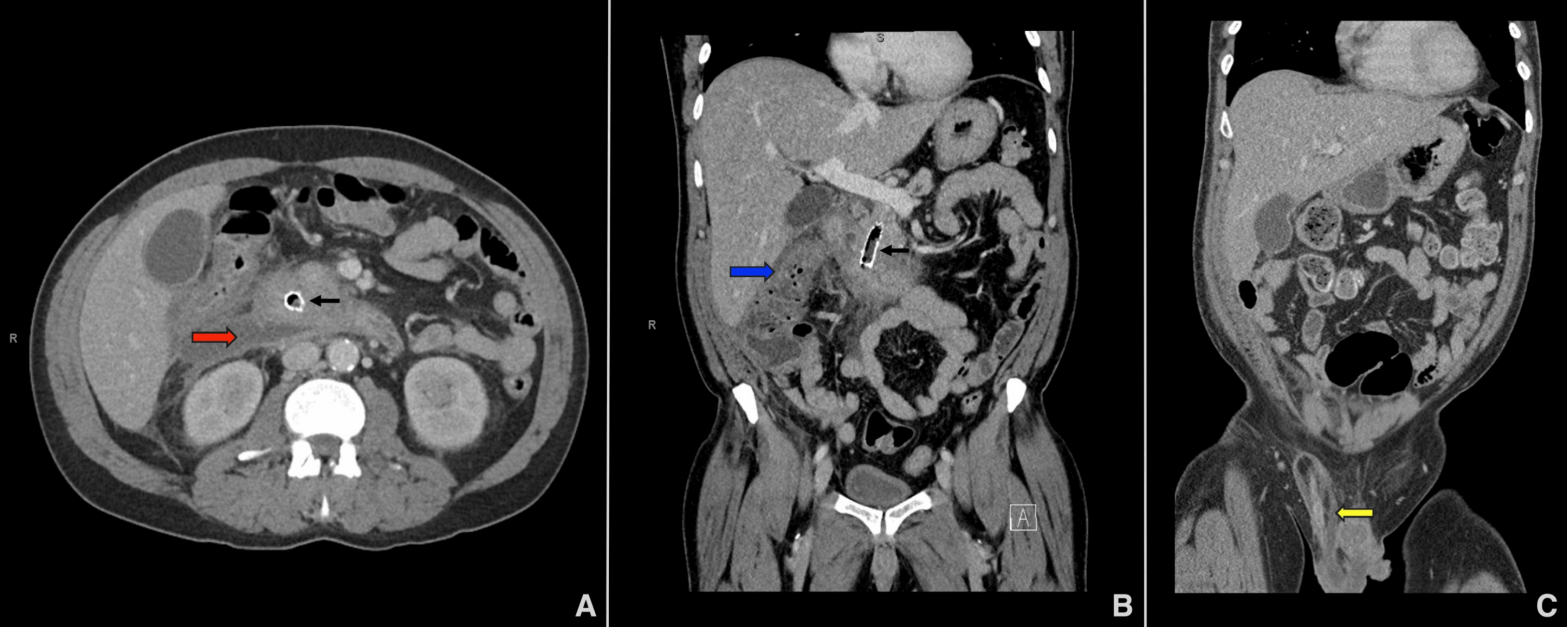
Multiple computed tomography scans revealed extensive abscesses. (A) Infected peripancreatic acute necrotic collection measuring approximately 2.5 × 7.5 × 8.5 cm (red arrow). (B) Contiguous inflammatory changes, including duodenitis and colitis at the hepatic flexure (blue arrow), extending toward the cecum and right hemiscrotum, with a fluid collection measuring approximately 9.8 × 2.9 cm. (C) Abscess formation within the right inguinal canal and spermatic cord (yellow arrow). The fully covered self-expandable metal stent (FCSEMS) is also visible (black arrows).
